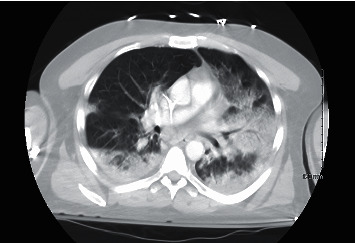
Computed tomography scan (CT) of the chest showing infiltrates in both lower lung lobes.
